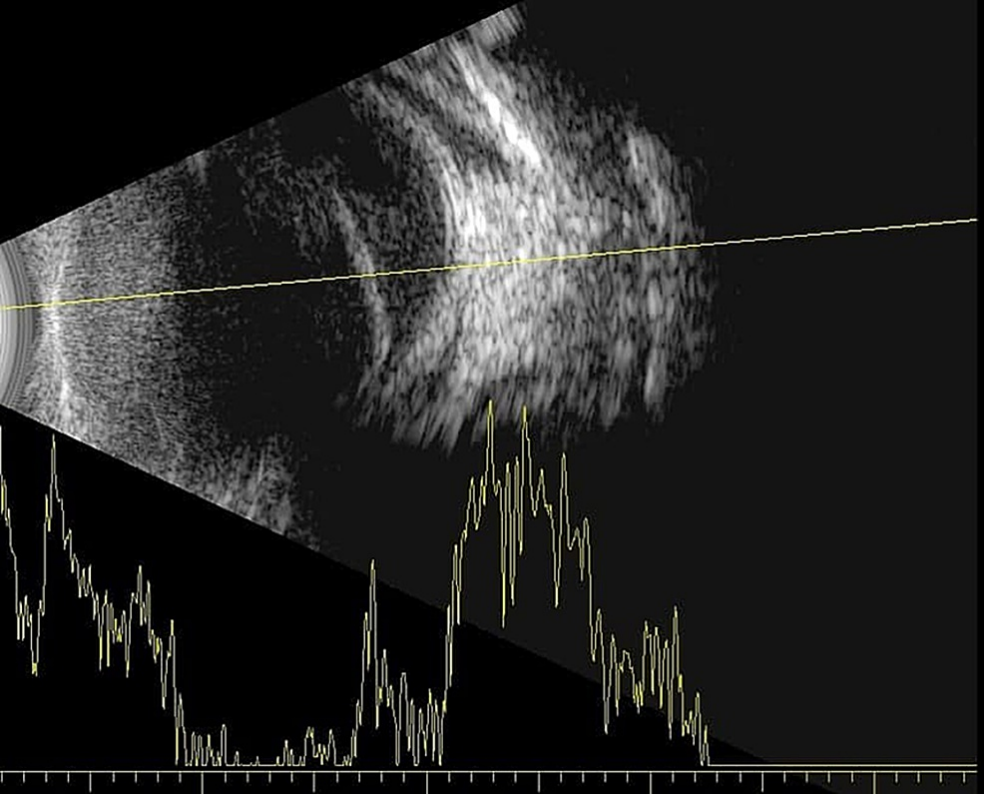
B-scan ultrasound picture of the left eye showing funnel-shape exudative retinal detachment.
